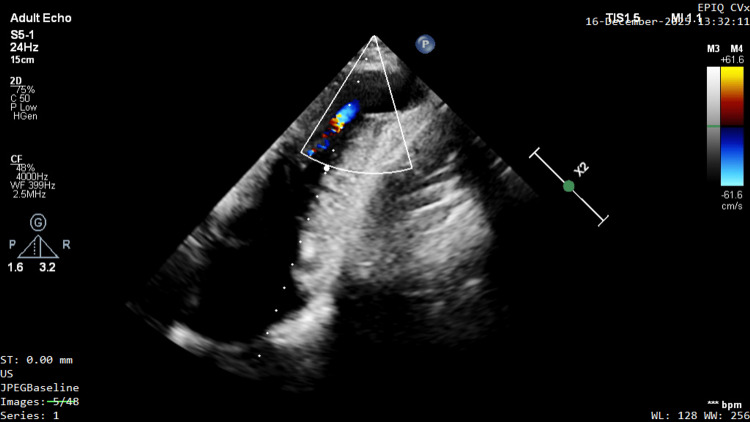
Following the absence of a visible aneurysm in the standard four-chamber view, detailed evaluation was performed in the apical two-chamber view. Meticulous intercostal "sliding" and long-axis "tilt" probe maneuvers successfully revealed an apical aneurysm connected to the previously noted color Doppler jet.
